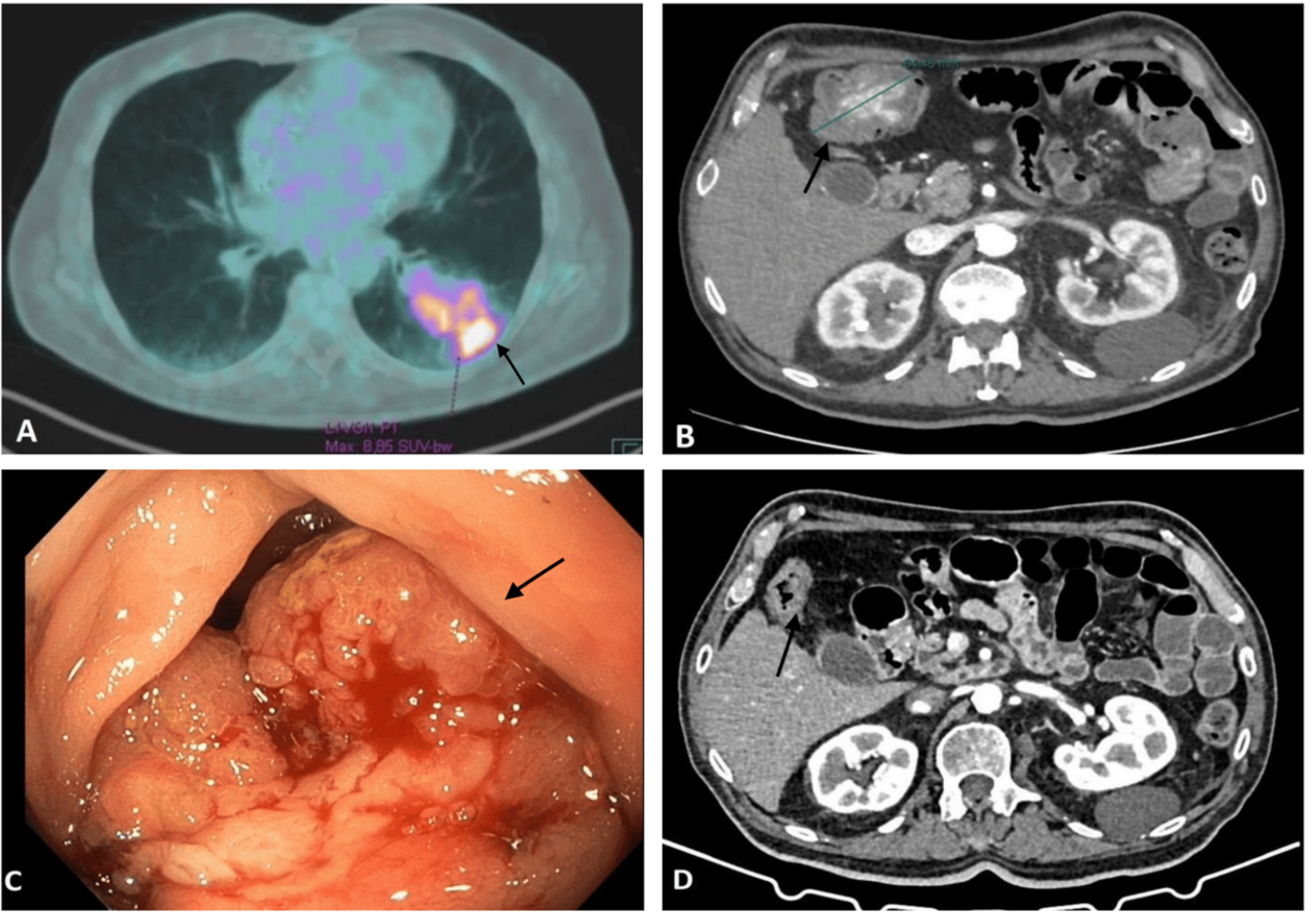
Radiological examination and endoscopic procedure. (A) PET scan showing the primary tumor before surgical resection (July 2021). (B-C) CT scan and colonoscopy showing the appearance of large bleeding colic metastasis (March 2022). (D) CT scan showing complete response of colonic metastasis after treatment with selpercatinib (September 2022).
